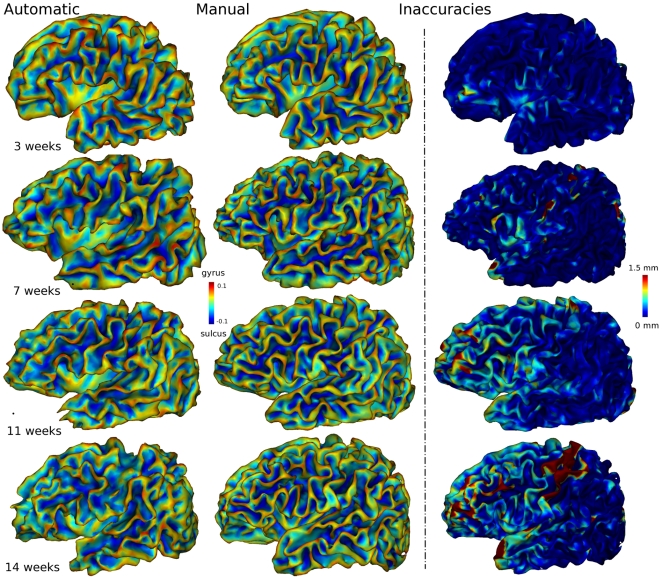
Validation of automatic segmentations against manual segmentations in 4 left hemispheres from infants with increasing ages (3 to 14 weeks). The two left columns show 3D renderings of the cortical surface reconstruction for automatic and manual segmentations. Color outlines surface curvature: gyri *in red* and sulci *in blue*. Method inaccuracy, i.e., distance from automatic to manual segmentation, is shown in the right column. Color map shows accuracy *in blue* and inaccuracy *in red* (in mm, up to 1.5 mm). Thus, *yellow-red* areas in the right column point to locations of segmentation inaccuracy. Inaccuracy patterns can further be analyzed in the two left columns by comparing the automatic surface to the manual one at those locations. See also in figure 1 for MRI slice samples of these four babies.
